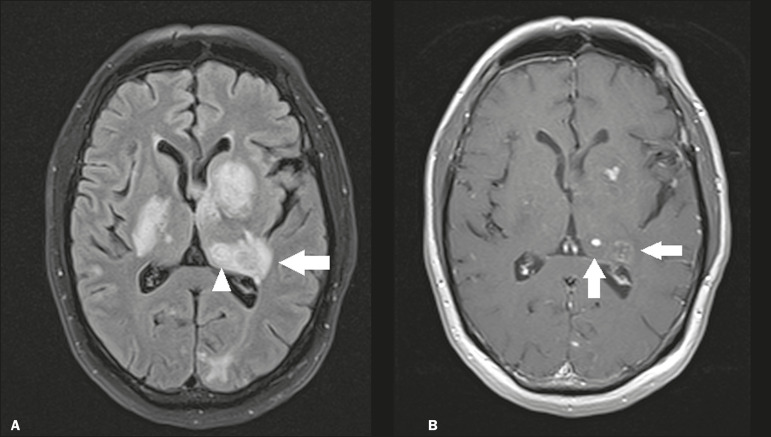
Neurotoxoplasmosis in a patient with acquired immunodeficiency syndrome who had a CD4 cell count of 126 cells/mm3. A: Axial FLAIR MRI sequence showing multiple hyperintense lesions in the nucleocapsular regions and in the left thalamus (arrow), with the eccentric target sign in the most medial lesion in the left thalamus (arrowhead). B: Contrast-enhanced axial T1-weighted MRI sequence showing nodular peripheral enhancement of the thalamic lesions (arrows).
